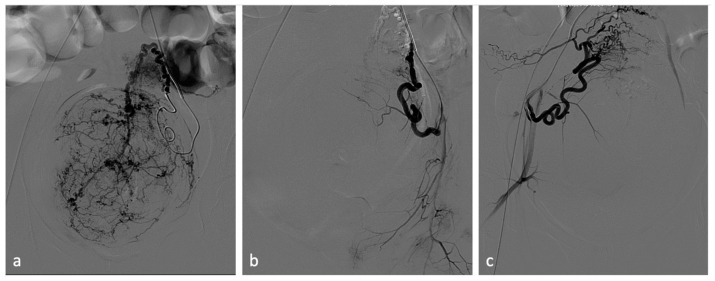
Uterine artery embolization for leiomyoma. A 27-year-old patient presenting with menometrorrhagias and pelvic pain. TVUS and MRI demonstrated a voluminous isthmic uterine leiomyoma (12 cm of diameter). Selective left uterine artery arteriogram (a) shows multiple vessels feeding the large leiomyoma. Embolization through the release of embolizing microparticles was performed. Post-embolization left artery arteriogram (b) shows good devascularization of the leiomyoma. Post-embolization selective right uterine arteriogram (c) showed ectasia of the right tubal artery with origin of tubo-ovarian trunk for the ipsilateral annexal branches. Given the high risk of non-target embolization, embolization of the right uterine artery was not performed.
